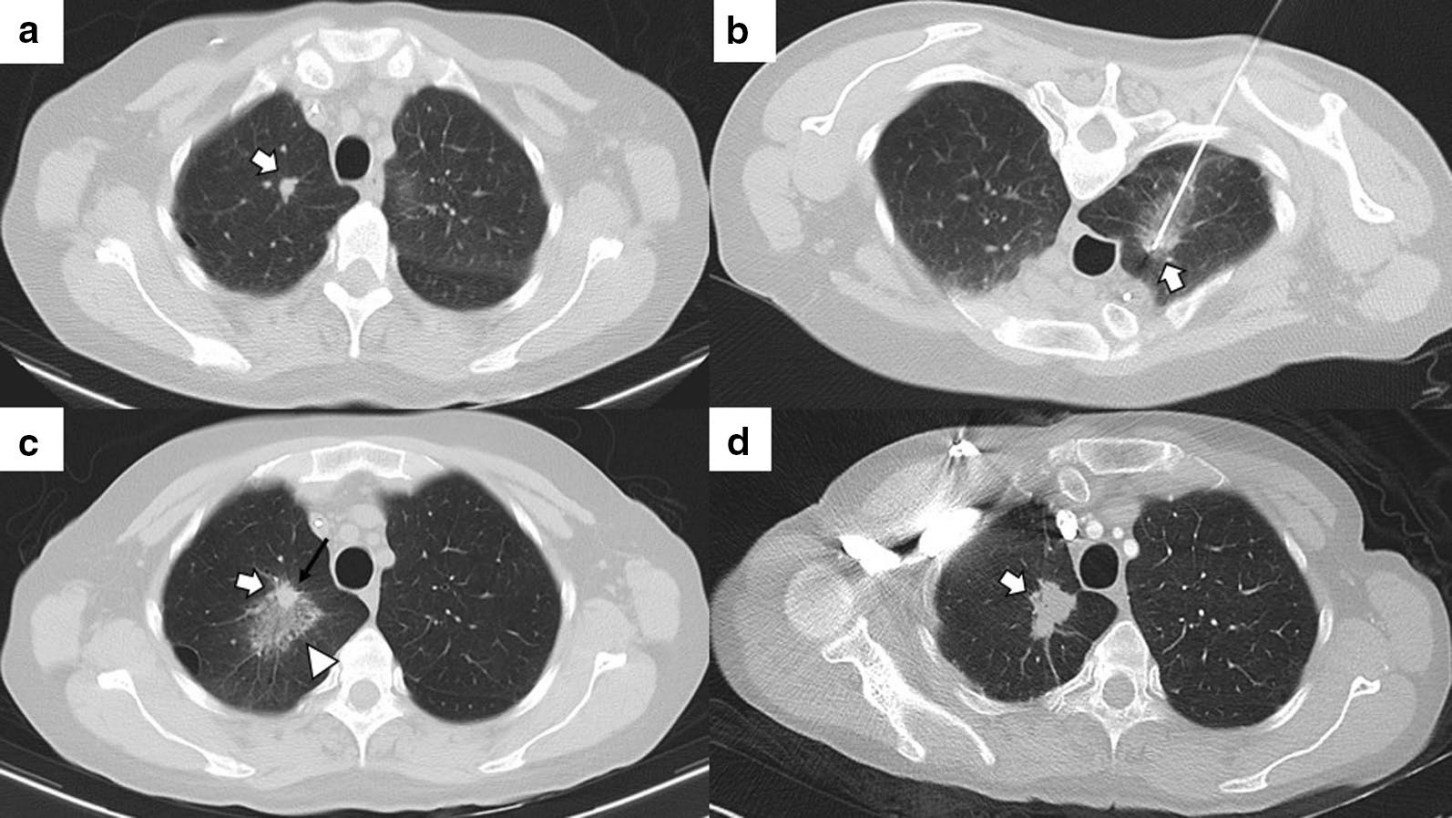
Tumor persistence after treatment with image-guided percutaneous lung ablation. a Non-enhanced CT showing a single metastatic tumor in the upper right lobe in a patient with rectal carcinoma. b CT-guided RFA treatment using a straight probe, passing through the center of the tumor. c A non-enhanced CT was performed 24 h after treatment, showing the treated lesion (thick white arrow) surrounded by a ground-glass halo (triangle). However, the treated tumor's frontmost portion does not present a ground-glass halo (thin black arrow), which may correspond to incomplete treatment. d Contrast-enhanced CT scan performed three months after treatment shows a considerable increase in the treated lesion, which now presents nodular and irregular borders, consistent with tumor persistence
